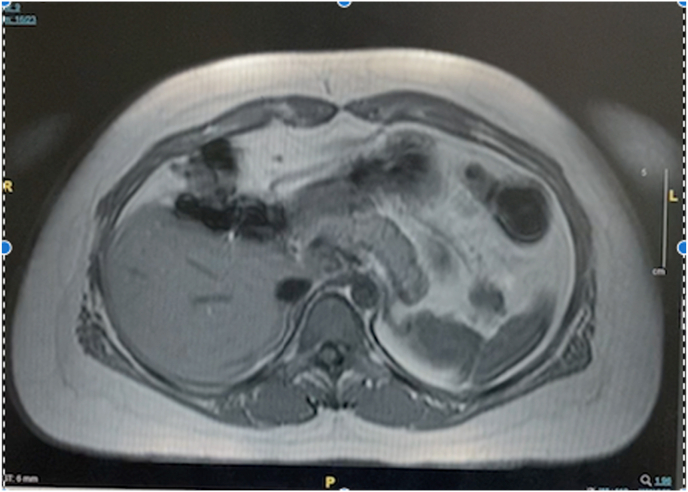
Magnetic Resonance Imaging (MRI) of the pancreas showing no obvious lesion in the pancreas.
